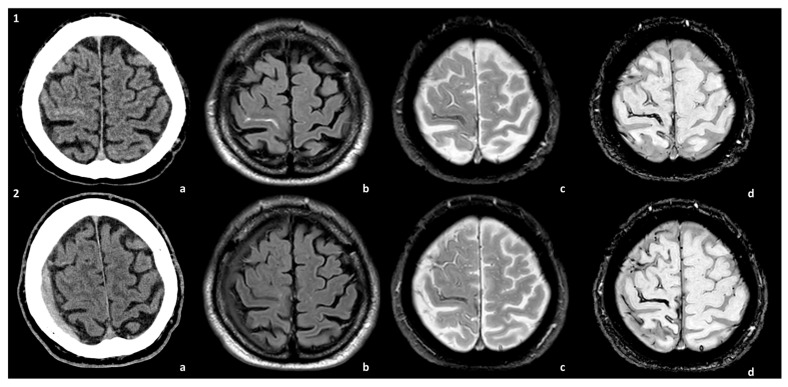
Spontaneous SDH in the natural history of a patient with CAA. The patient presented with TFNEs and convexal subarachnoid hemorrhage with cortical superficial siderosis (cSS) (panel 1). A right hemispheric SDH was found in the control MRI at 1 year (panel 2). The images are compared at the two time points of the natural history of the patient: (a) non-contrast CT, (b) axial Fluid-Attenuated Inversion Recovery (FLAIR) sequence, (c) T2* sequence, and (d) Susceptibility-Weighted Imaging (SWI) sequence.
